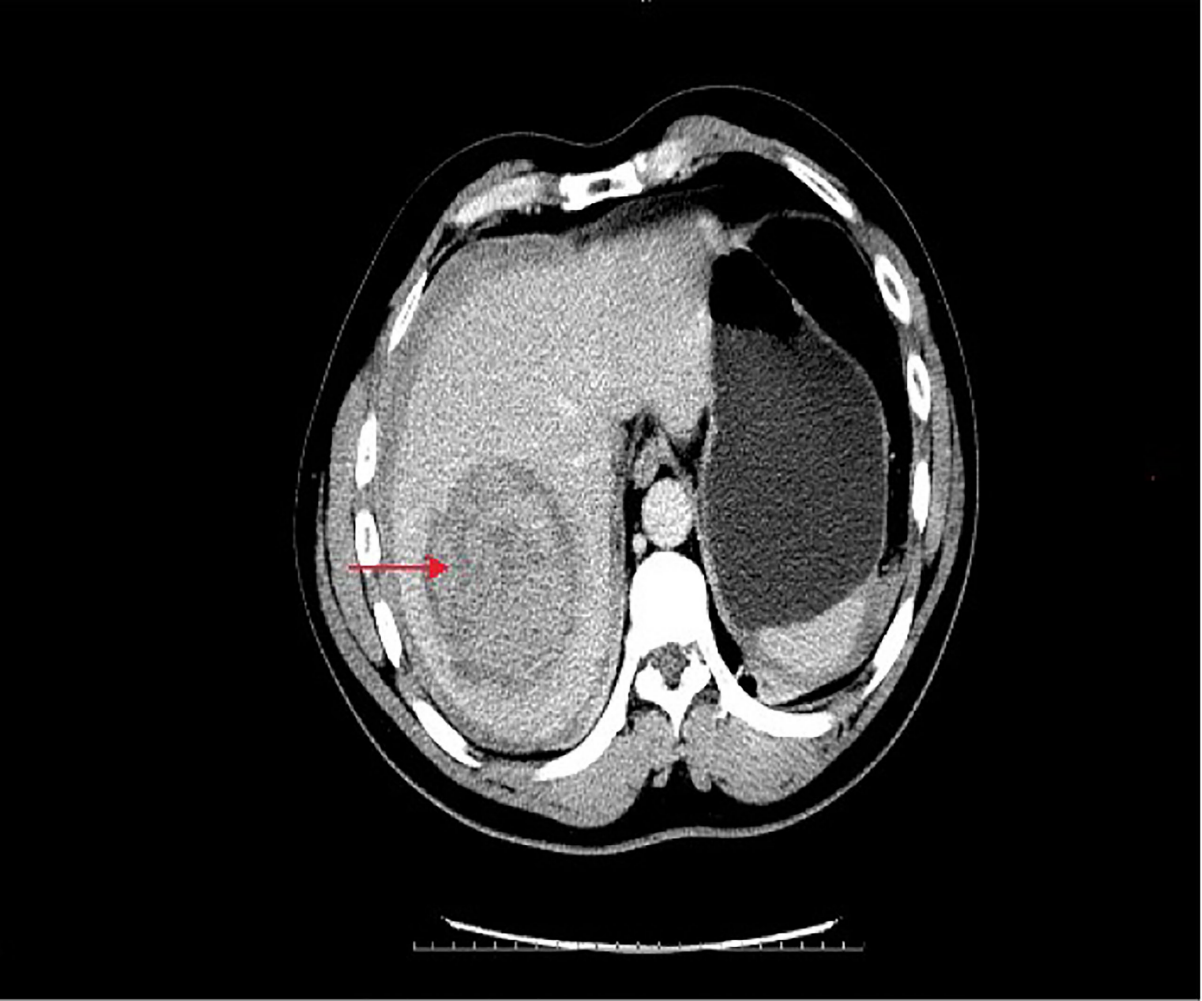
Emergency CT of the abdomen showed a mixed density mass of about 8*8 cm in the right lobe of the liver. The lesion was near the right hepatic artery and free liquid density was visible around it.
